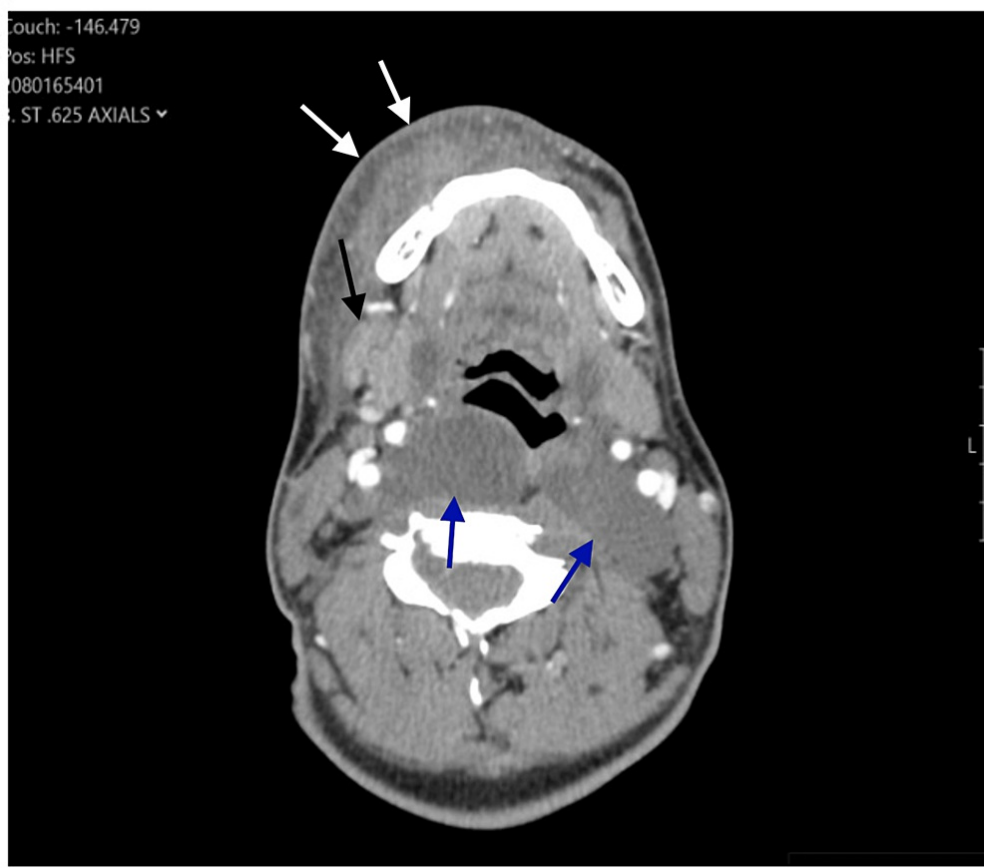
CT scan of the neck's soft tissues with contrast. White arrows: Skin thickening, inflammatory changes, and edema along the subcutaneous tissue of the right lower lip.
